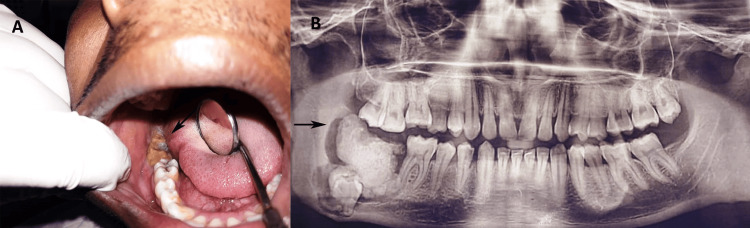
Clinical and radiological features (A) bony, hard yellowish-brown mass distal to the first molar on the right side of the mandible. (B) The OPG shows a radiopaque mass surrounded by a radiolucent rim and is associated with an impacted second molar at the lower border of the mandible. OPG: orthopantomogram
